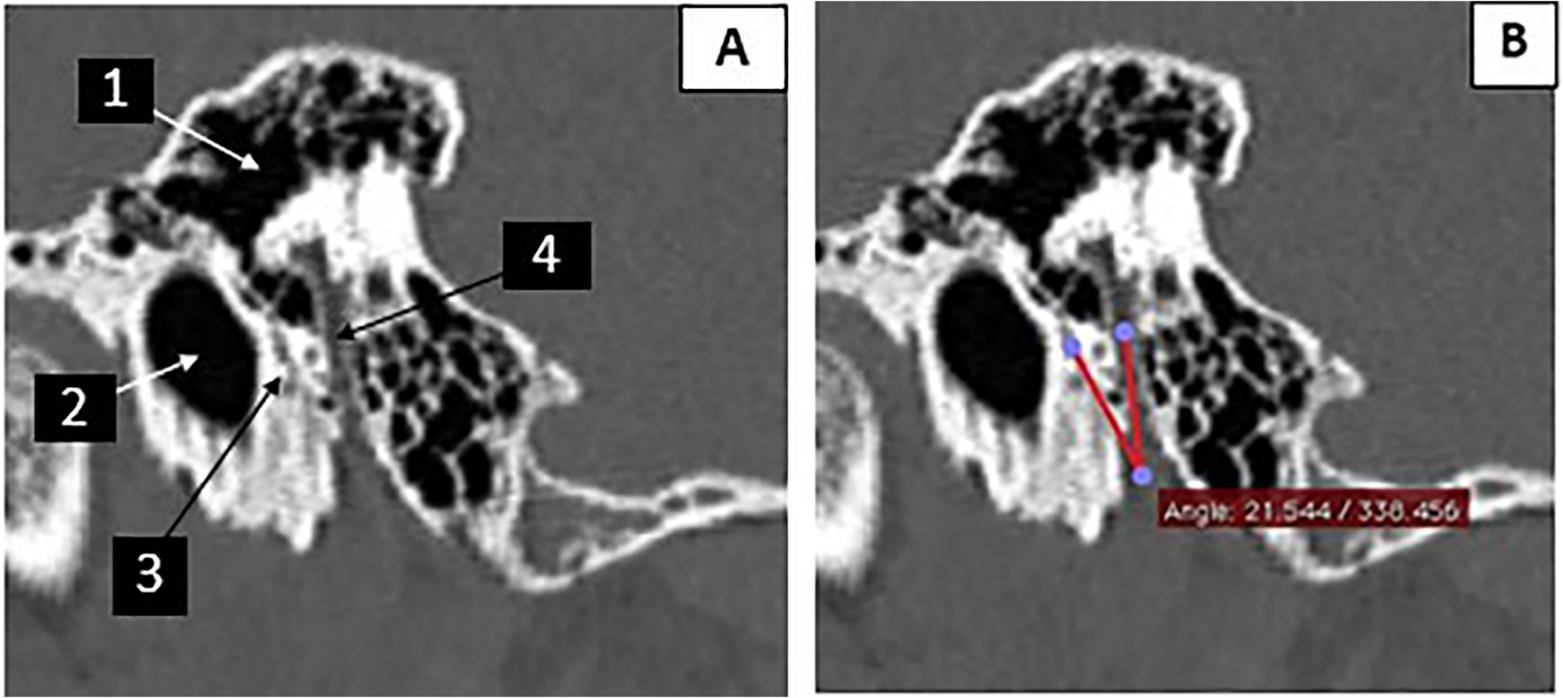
The image of case number 233 (male patient aged 3.2 years). A The parasagittal view of the preoperative HRCT. (1 = the middle ear, 2 = the external auditory canal, 3 = the chorda tympani nerve, 4 = the mastoid portion of the facial nerve). B the chorda-facial angle = 21.5°
